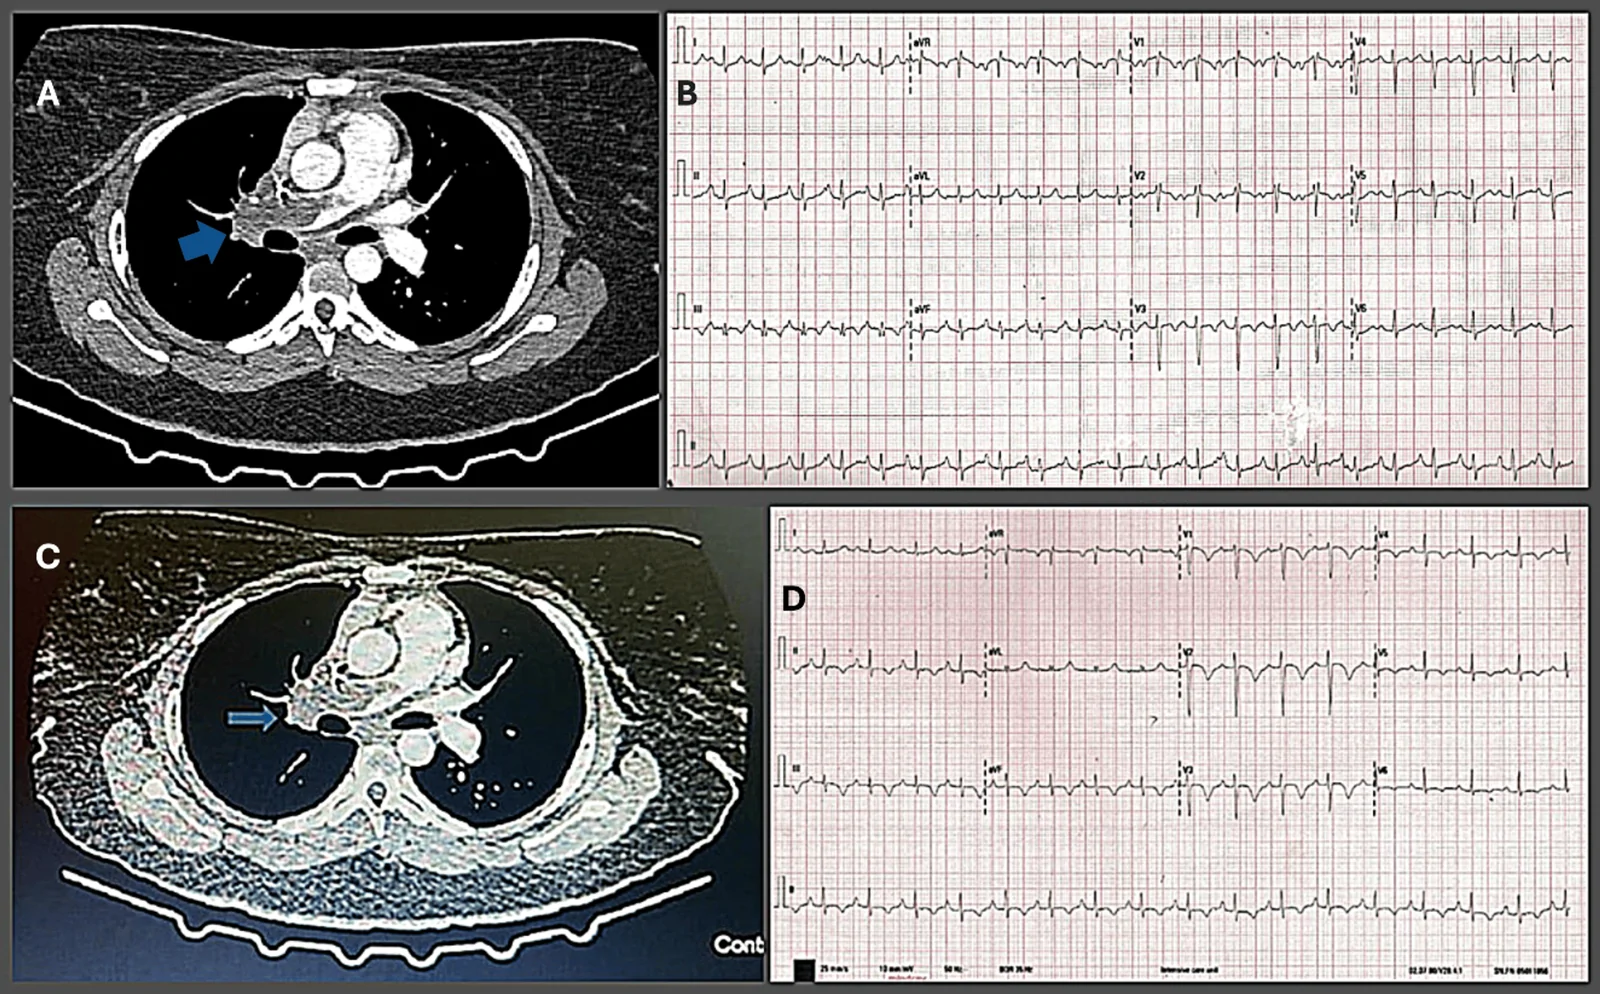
Case 6: A. Pre-thrombolysis chest CT angiogram shows the right main pulmonary artery thrombus (blue arrows). B. Pre-thrombolysis shows sinus tachycardia and incomplete right bundle branch block (RBBB). C. Post-thrombolysis chest CT angiogram shows minimal resolution of the right main pulmonary artery thrombus (blue arrow). D. Improved tachycardia with resolution of RBBB and persistent widespread T-wave abnormality.
